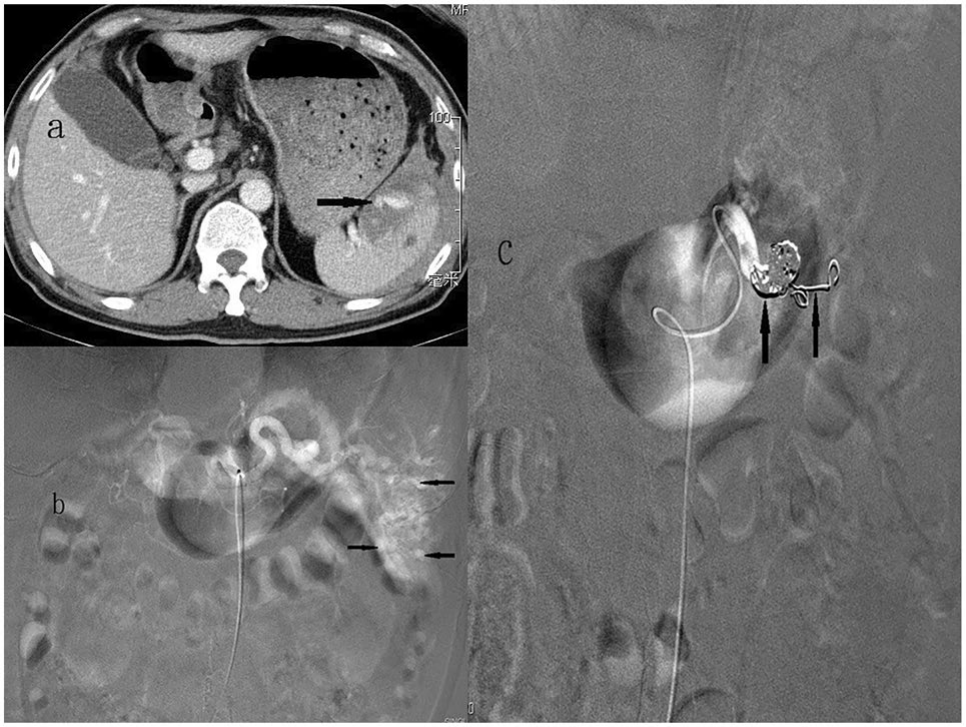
58-Year-old man with a grade IV blunt splenic injury underwent proximal splenic arterial embolization. a Admission contrast-enhanced helical computed tomography scan demonstrates a low attenuation laceration with a big pseudoaneurysm (arrow) and perisplenic hematoma. b The splenic angiogram demonstrates multiple pseudoaneurysms (arrows) at lower half of spleen. c The post-embolization angiogram demonstrates the deployment of metallic coils in the main splenic artery (arrows). Pseudoaneurysm is no longer present
